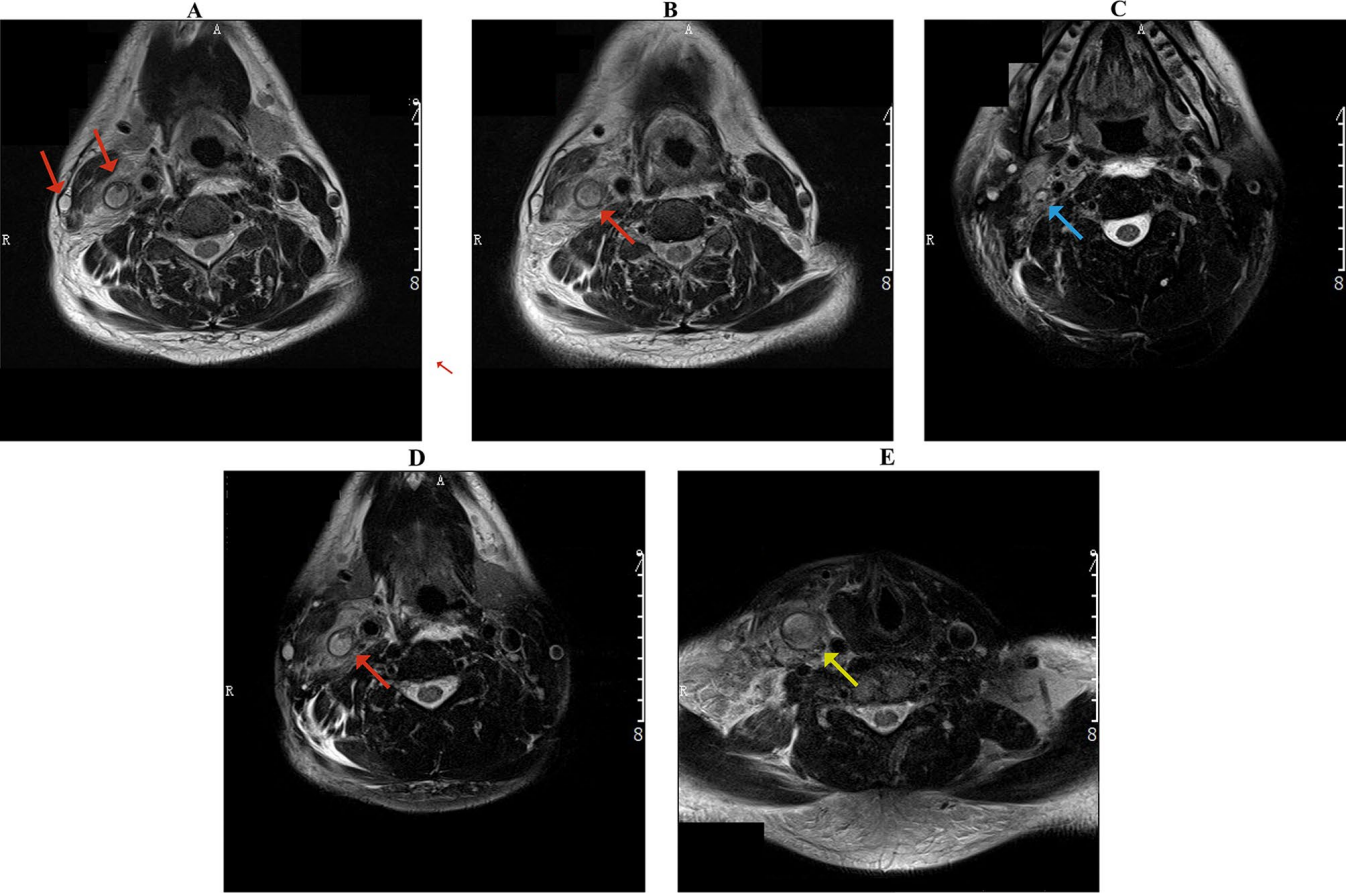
Cervical contrast-enhanced MRI (July 13, 2020). Axial images (A-E) show complex lesions: thrombosis of the right internal jugular, external jugular, and subclavian veins (A, B, D; red arrows), inflammatory changes in the right parapharyngeal space (C; red arrow), and multiple enlarged lymph nodes with clavicle osteomyelitis in the right supraclavicular fossa (E; red arrow)
